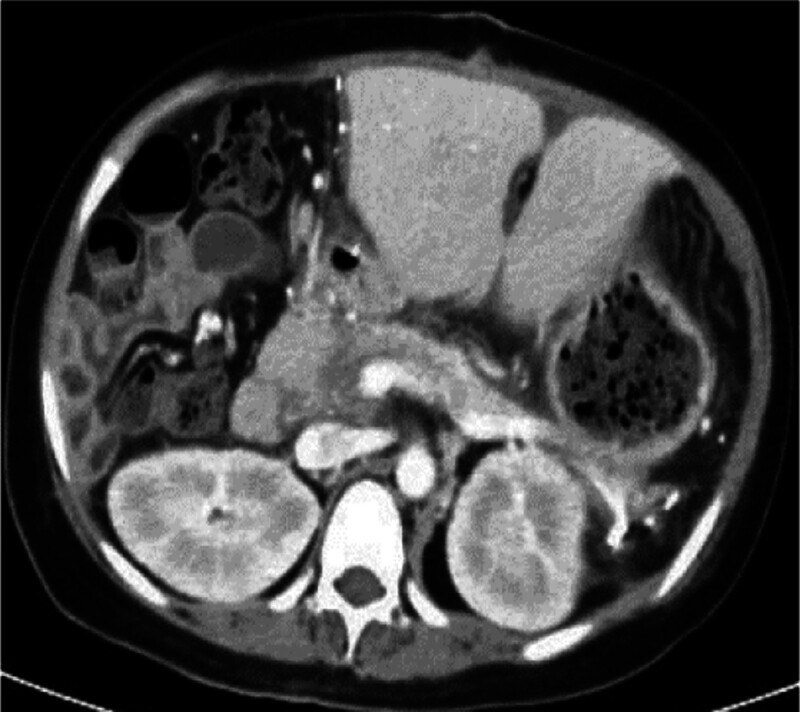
Enhanced CT scans of the upper abdomen half a month and 2 and a half months after the operation, respectively. The pancreas shrinks, its boundary is clear, and its blood vessels decrease, especially in the head of the pancreas. Ischemic infarcted area without enhancement is seen in the head of the pancreas (black arrow). CT = computed tomography.
